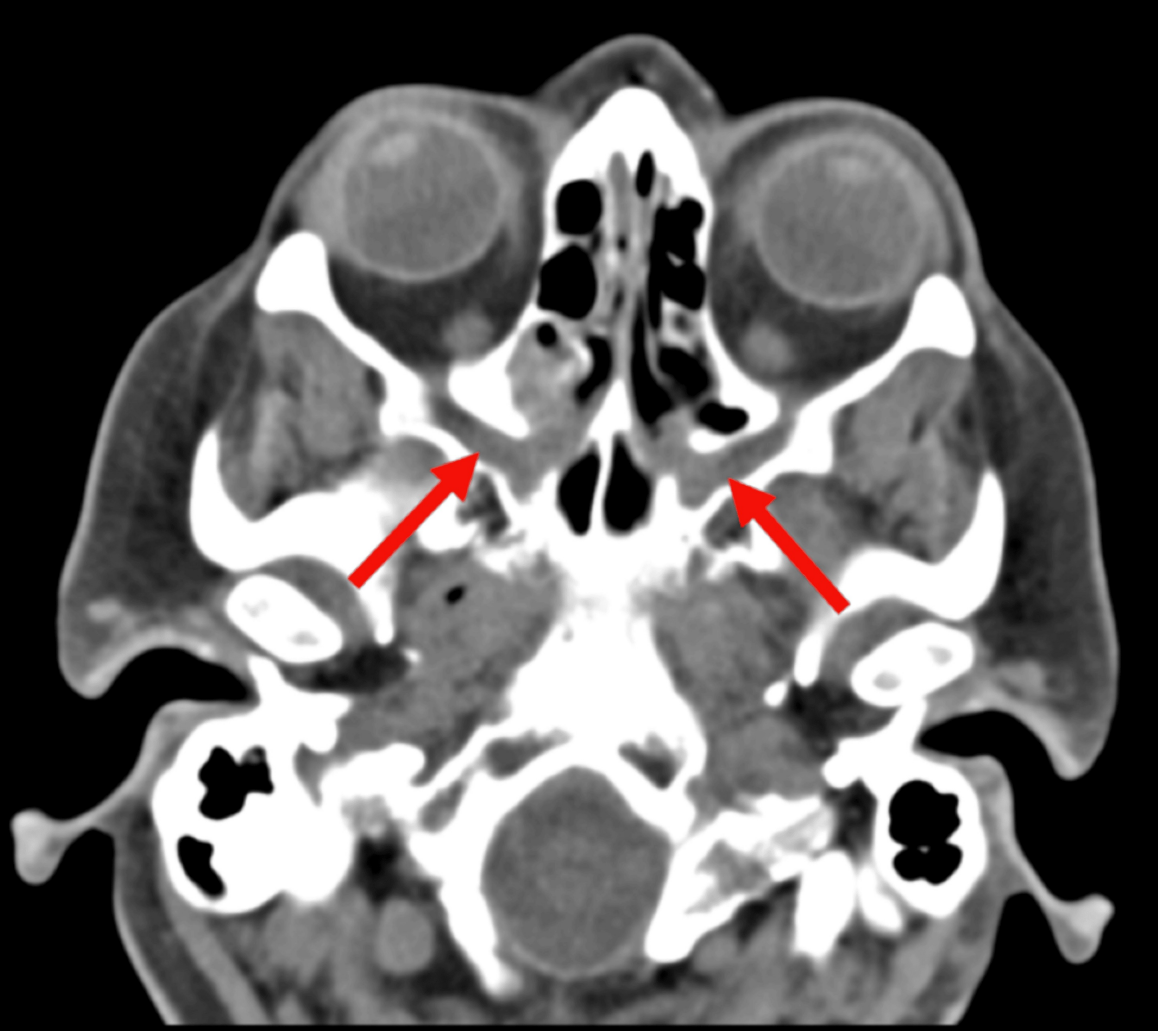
Axial head CT with IV contrast demonstrating abnormal soft tissue thickening in the pterygopalatine fossa (arrows), extending into the sphenopalatine foramen and involving the pterygoid canal, concerning for an infiltrative process. CT, Computed Tomography
